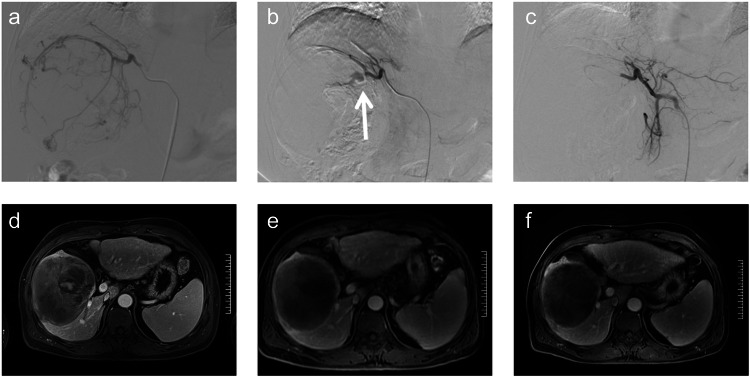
Images in a 59-year-old man with advanced hepatocellular carcinoma. a Hepatic angiography during the first TACE. b In the first DEB-TACE, CalliSpheres@ Beads with a diameter of 100-300 um loaded with 60 mg of doxorubicin were used to embolize the right hepatic artery, and the VL phenomenon occurred in the re-examination angiography (arrow). c PVA particles with a diameter of 350-560 μm were subsequently added for embolization until the VL phenomenon disappeared in the re-examination angiography. d Enhanced MRI at 4 weeks after the first DEB-TACE revealed partial contrast enhancement in the peripheral intrahepatic lesion, suggesting a partial response according to mRECIST criteria. Each small division on the scale bar represents 1 cm. e After 3 rounds of DEB-TACE plus apatinib, MR images at 6 months of follow-up show that the tumor had a complete response. Each small division on the scale bar represents 1 cm. f One-year follow-up MR images show that the tumor had a complete response. Each small division on the scale bar represents 1 cm
